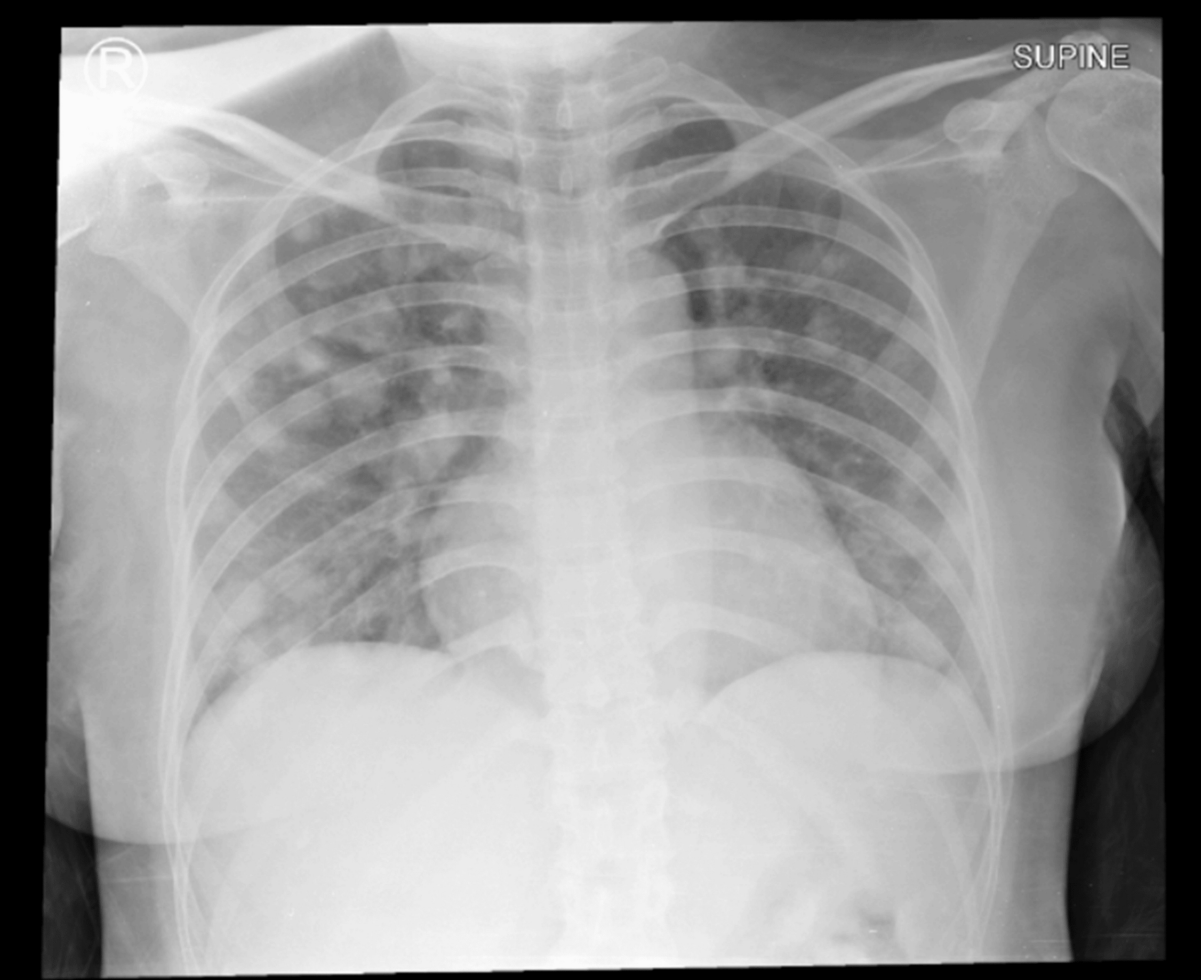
Chest X-ray of the patient showing multiple lung nodules.
